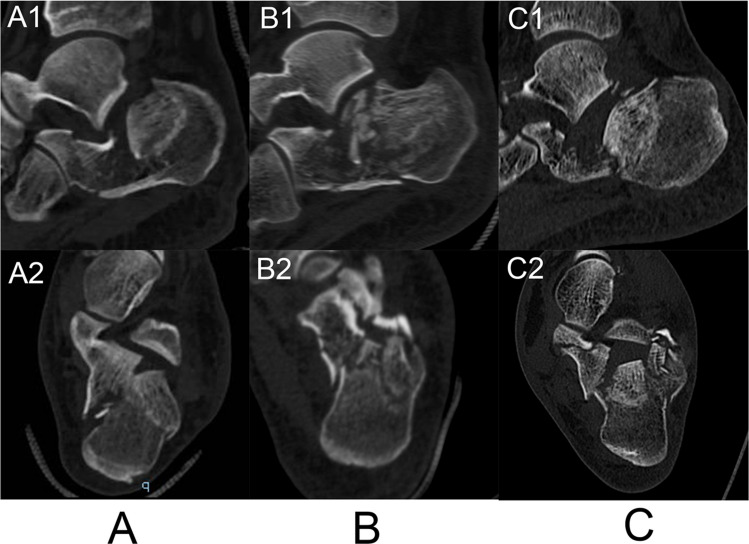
Intact tuberosity fragment in joint depression-type fractures. A–C Sanders types II, III, and IV, respectively. (A1, B1, C1) Sagittal CT scans. (A2, B2, C2) Coronal CT scans. CT, computed tomography
